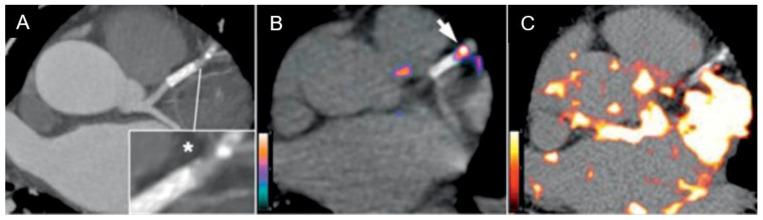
Comparison between CTCA with culprit stenosis (star in the pop out box) in the left anterior descending artery (A), ^68^Ga DOTATATE showing localized accumulation (arrow) corresponding to macrophage density and inflammation (B), and ^18^F FDG with more subtle focal accumulation confounded by high background accumulation (C). Adapted (cropped) from Tarkin et al. [17]. 2017 Copyright the Authors.
